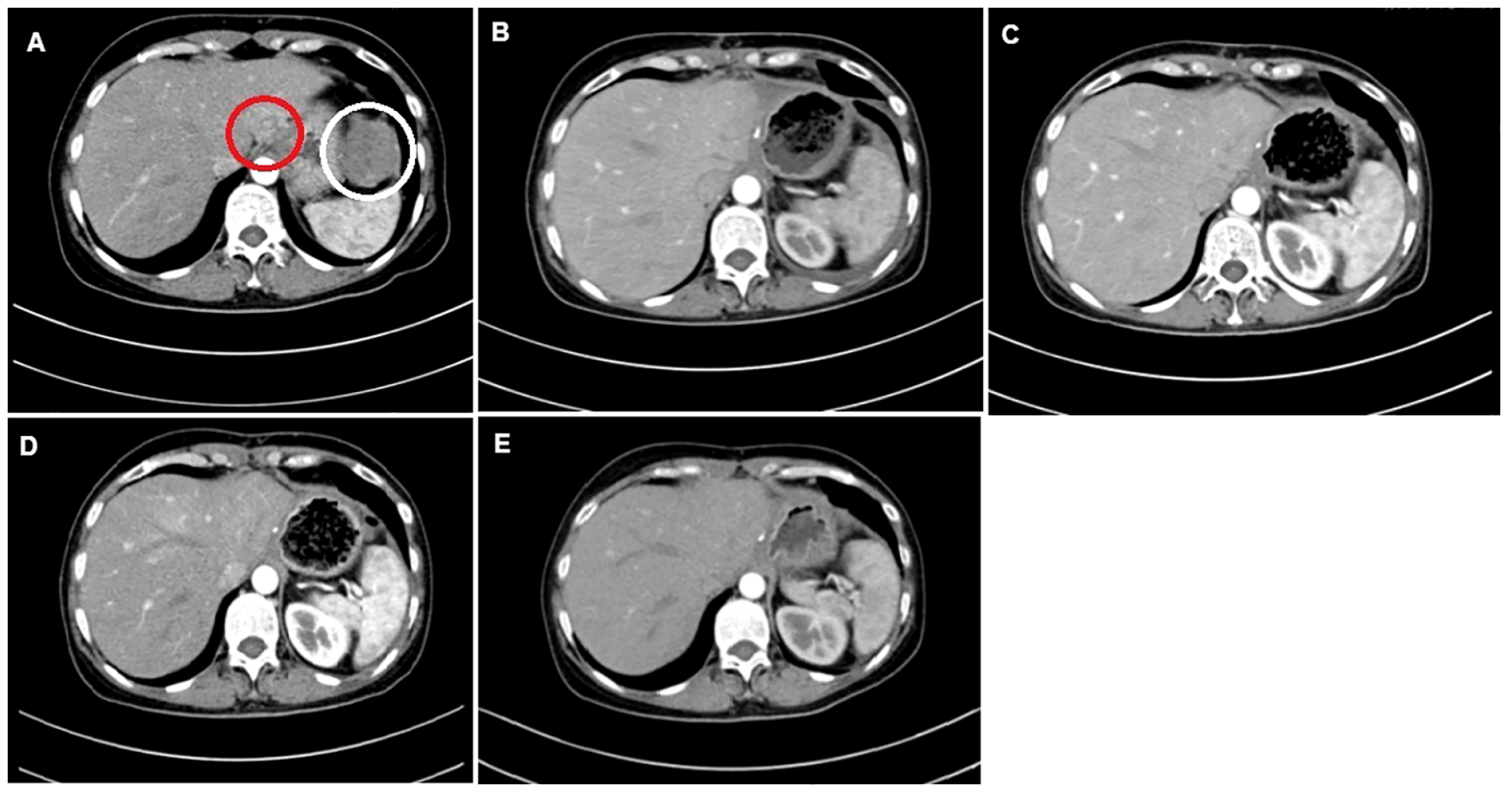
CT images of the 53-year-old female patient. (A) Tumor lesions of size 4.9 × 3.6 cm (red circle) and 7 × 6 cm (white circle). (B) Post-operative CT image before the first cycle of the GS regimen. (C) CT image post-chemotherapy after the second cycle of the GS regimen. (D) CT evaluation post-chemotherapy after the fifth cycle of the GS regimen. (E) CT evaluation post-chemotherapy after the sixth cycle.
